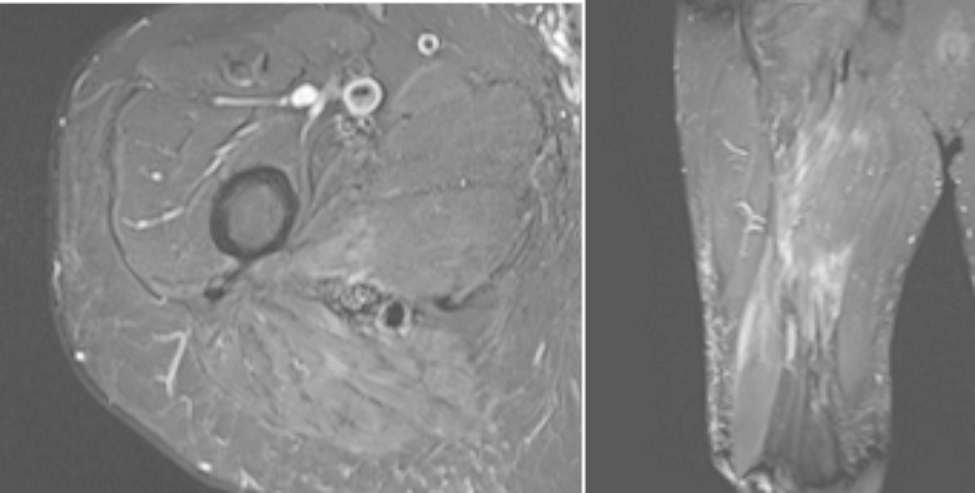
Post-recurrence postoperative T2-weighted MRI (left: axial; right: coronal)
